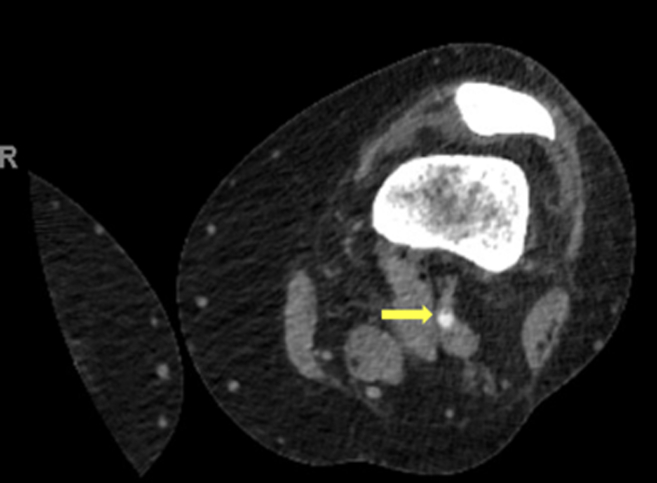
Computed tomography angiography with contrast demonstrates a nonobstructive dissection flap in the left popliteal artery at the level of the distal femoral metaphysis (arrow).
